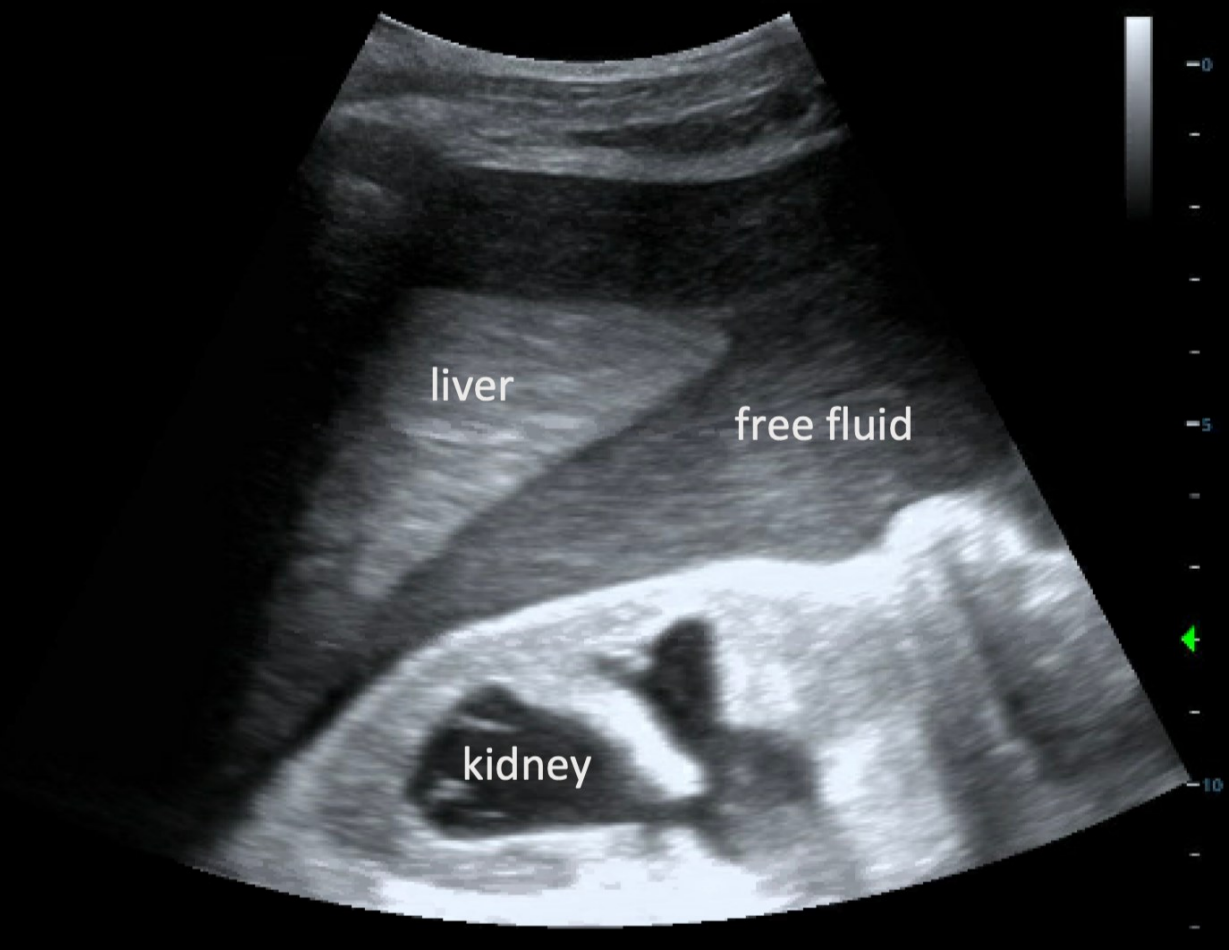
Point of care ultrasound in sagittal plane demonstrating large amount of free fluid in Morison’s pouch with moderate right-sided hydronephrosis.
